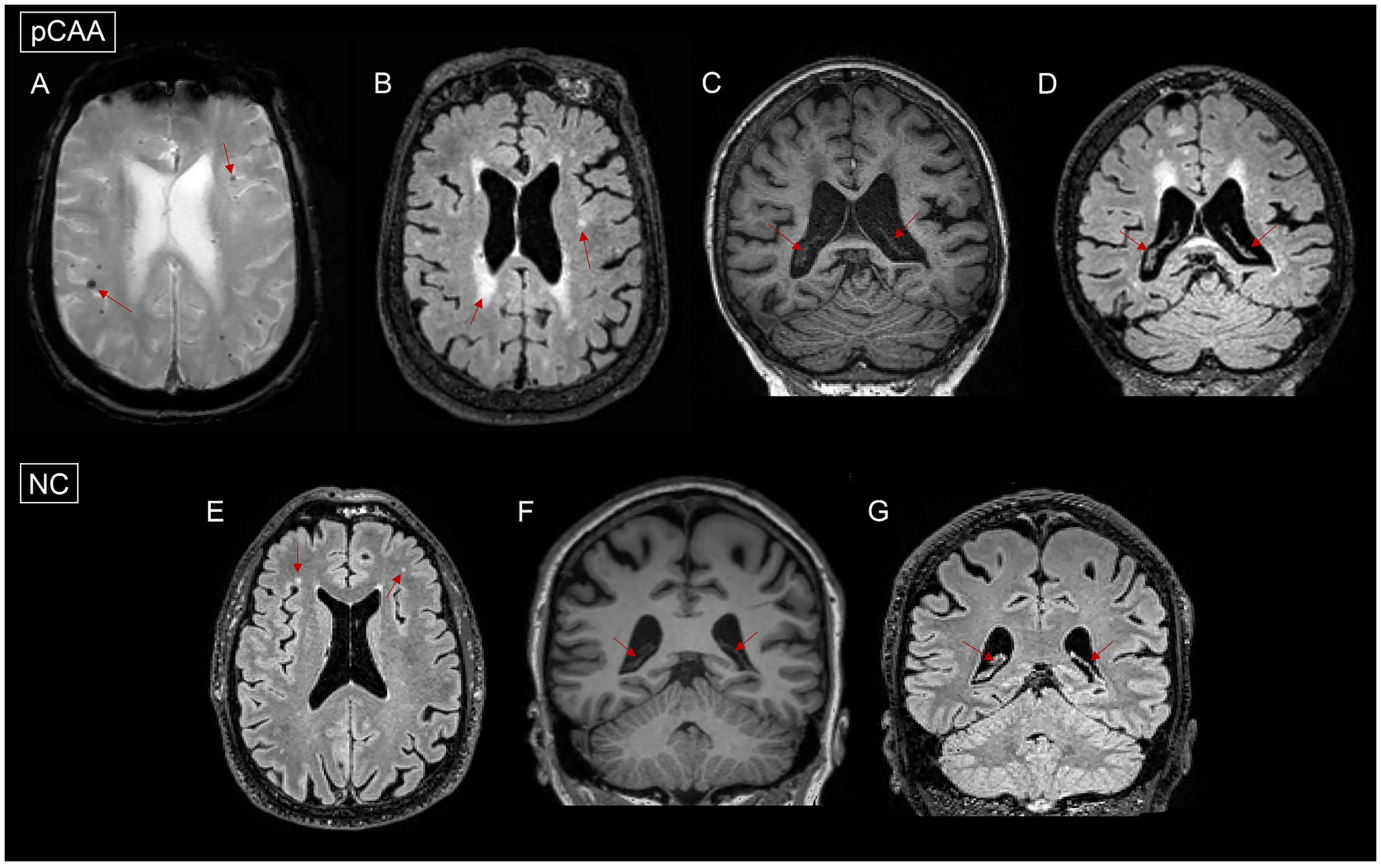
Images of microbleeds, white matter hyperintensities (WMH), and choroid plexus (ChP) in a normal control (NC) and a patient with probable cerebral amyloid angiopathy (pCAA). (A): pCAA demonstrates microbleeds on T2*-weighted sequences. (B): pCAA demonstrates white matter hyperintensities on T2 FLAIR sequences. (C): pCAA demonstrates ChP on T1-weighted sequences. (D): pCAA demonstrates ChP on T2 FLAIR sequences. (E): NC demonstrates white matter hyperintensities on T2 FLAIR sequences. (F): NC demonstrates ChP on T1-weighted sequences. (G): NC demonstrates ChP on T2 FLAIR sequences.
